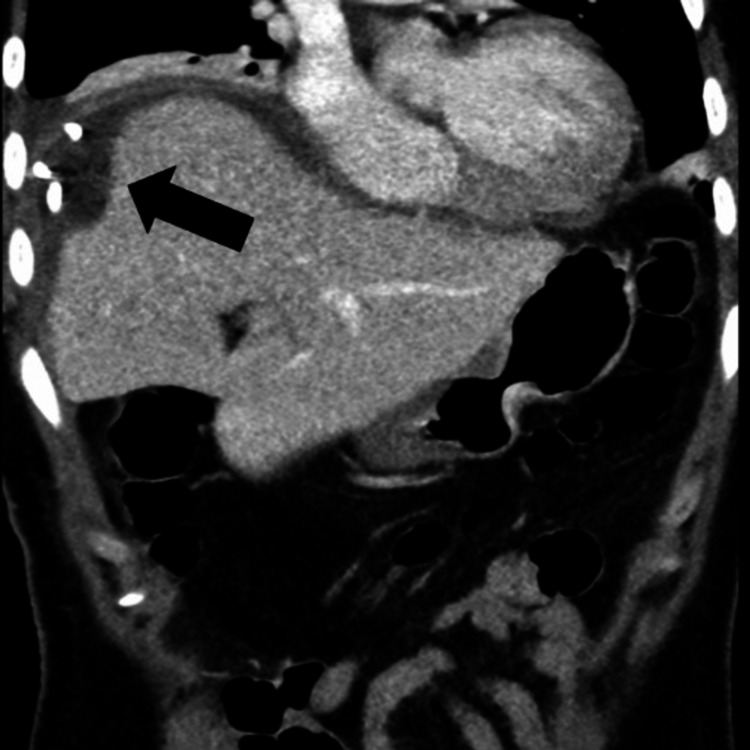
CT abdomen and pelvis, coronal image, shows interval placement of a percutaneous drainage catheter and decrease in size of the peri-hepatic collection (arrow).
